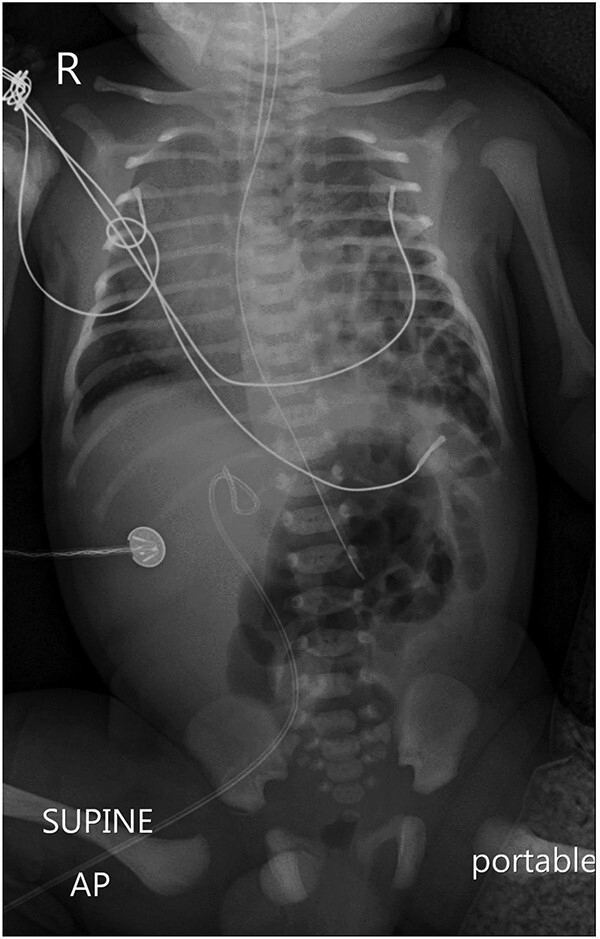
A portable X-ray photo of the chest, abdomen and pelvis shows the right mediastinal shift, total collapse of the left lung, partial collapse of the right lung and air-filled structures (bowel loops) extending from the abdomen into the chest.
